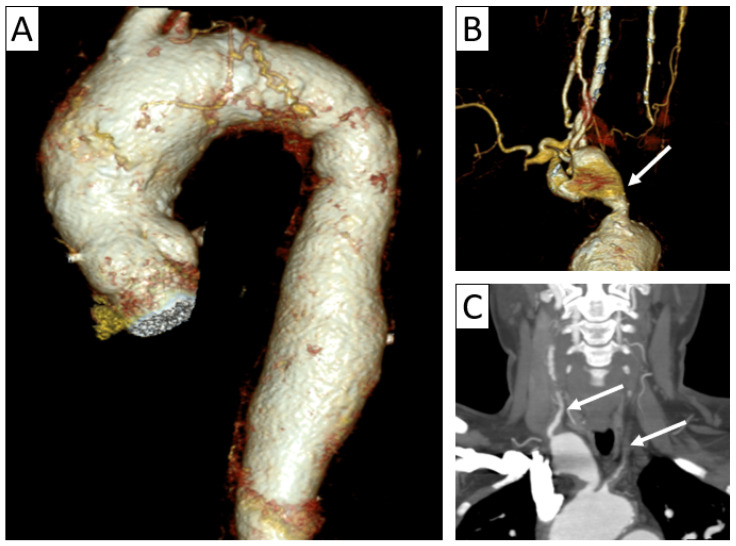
Complications of vasculitis—stenoses and aneurysms. Thoracic and supra-aortic trunk CTA with 3D volume rendering (A,B) and coronal reformatting (C) in a 41-year-old patient with TAK showing ectasias of the ascending and descending thoracic aorta (A), aneurysm of brachiocephalic artery (arrow in (B)) and stenoses of the common carotid arteries (arrows in (C)), as well as subclavian arteries.
